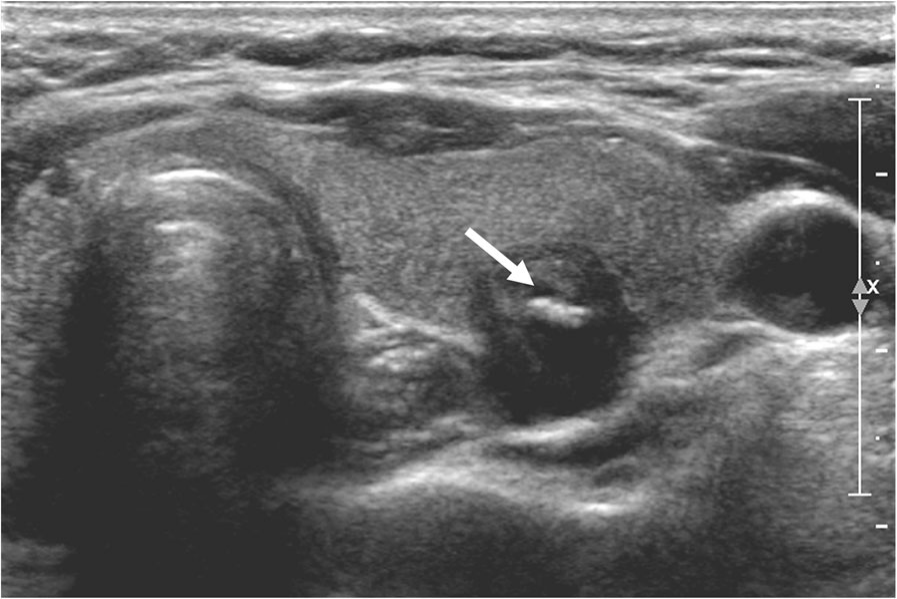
Echogenic foci associated with malignancy. 51-year-old female with thyroid nodule. Ultrasound image shows a 1.1 cm hypoechoic, solid nodule with large echogenic foci (> 1 mm) without shadowing (Type 3, arrow). Biopsy result was papillary carcinoma and was confirmed at surgery
